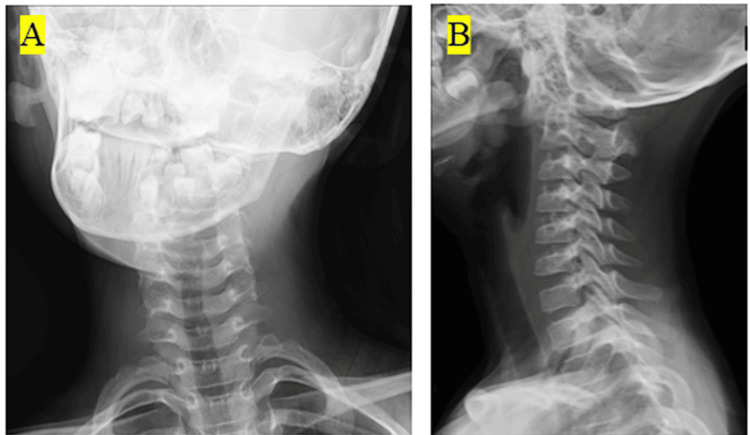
(A) Frontal X-ray image of the cervical spine. An oblique neck position was observed. (B) Lateral radiograph of the cervical spine. Lateral alignment of the cervical spine was intact. Image reproduced from Hashimoto et al. (2024) [14] reproduced under the Creative Commons Attribution (CC BY) license.
